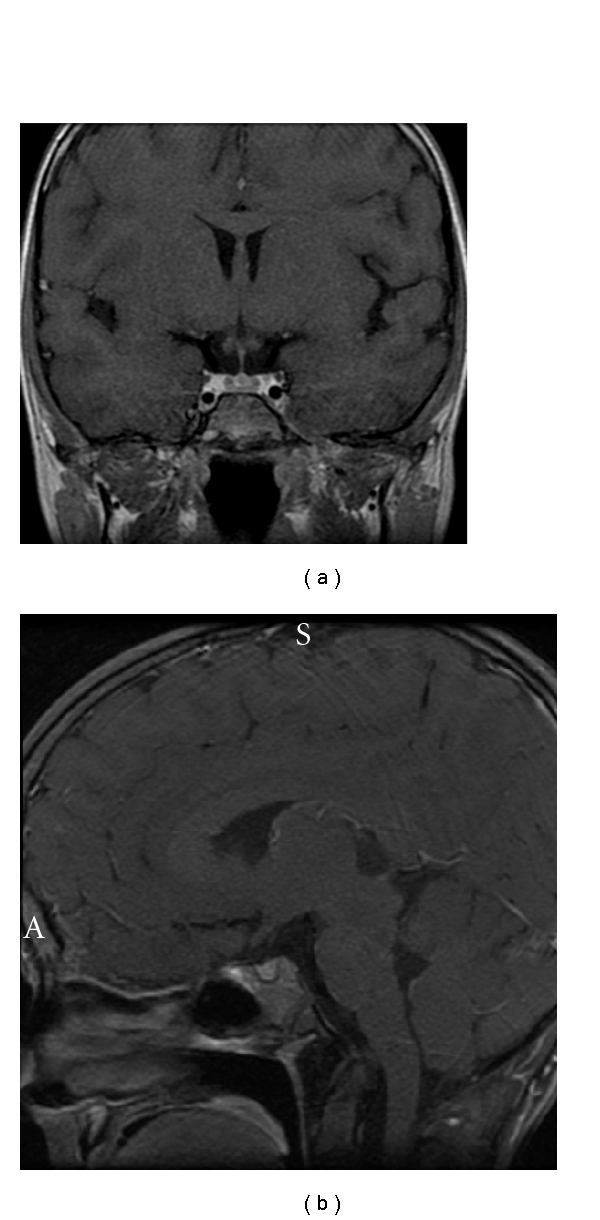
Magnetic resonance imaging scan of the brain. A coronal section shows a tiny cyst in the midline of the pituitary gland (a). A sagittal section demonstrates that the tiny cyst is at the posterior margin of the anterior pituitary (b).
